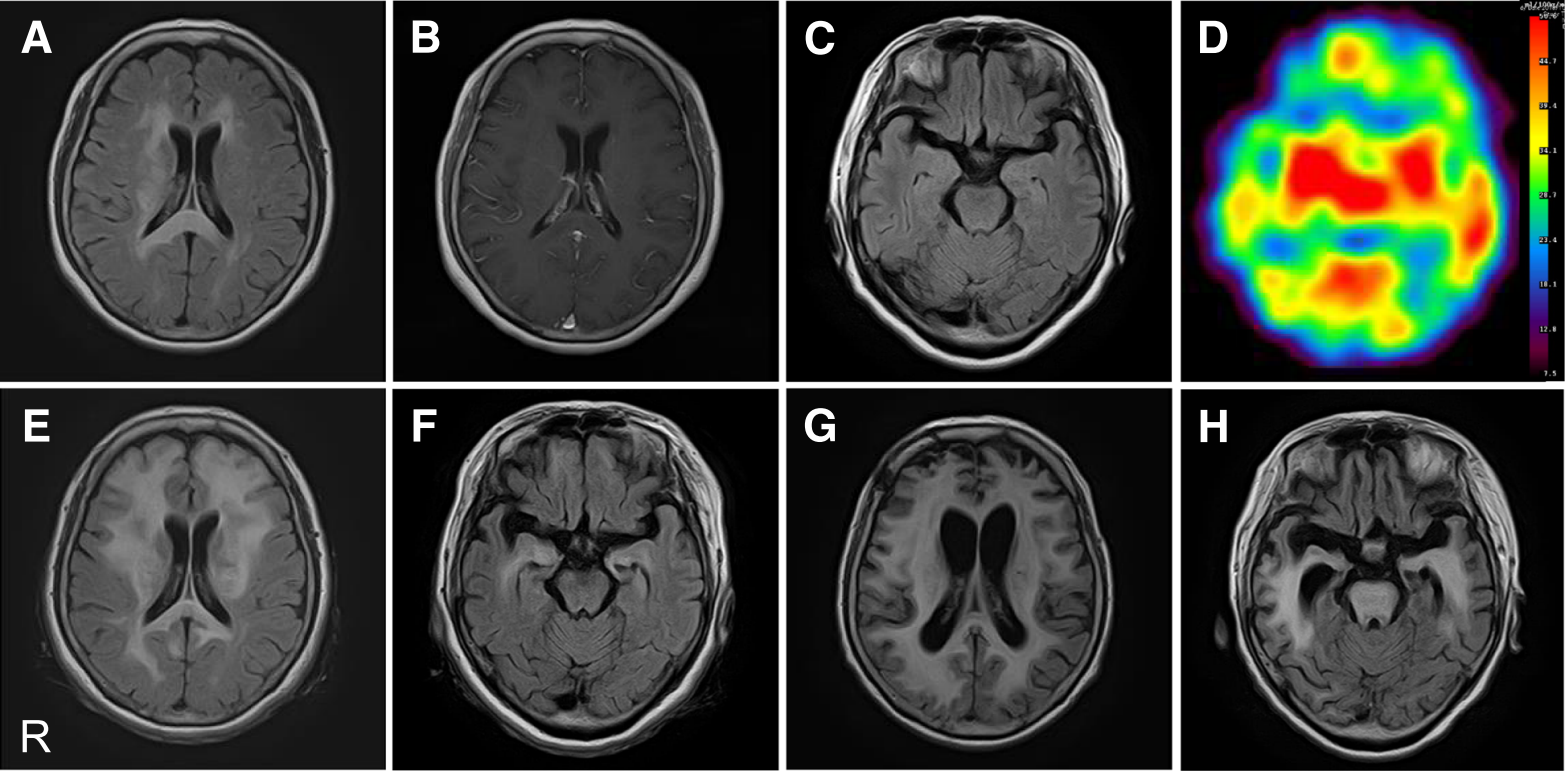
Brain magnetic resonance images and single photon emission computed tomography (SPECT). a-c Two months after fever onset, fluid-attenuated inversion recovery (FLAIR) images show diffuse high signal intensity in the splenium of the corpus callosum and right-hemisphere dominant subcortical white matters. However, this finding is (a) neither exhibited in the temporal lobes nor exhibited in the brainstem (c). No contrast enhanced lesions are observed in gadolinium-enhanced T1-weighted images (b). d SPECT study shows hyperperfusion in the basal ganglia, thalamus and brainstem. (E-H) Axial FLAIR images. Approximately five months after the onset of fever, hyperintensity lesions extended to the frontotemporal lobes, bilaterally (e, f). Twelve months after the onset of fever, FLAIR images show diffuse progression of infiltrative lesions in the whole brain including the brainstem with systemic brain atrophy (g, h)
